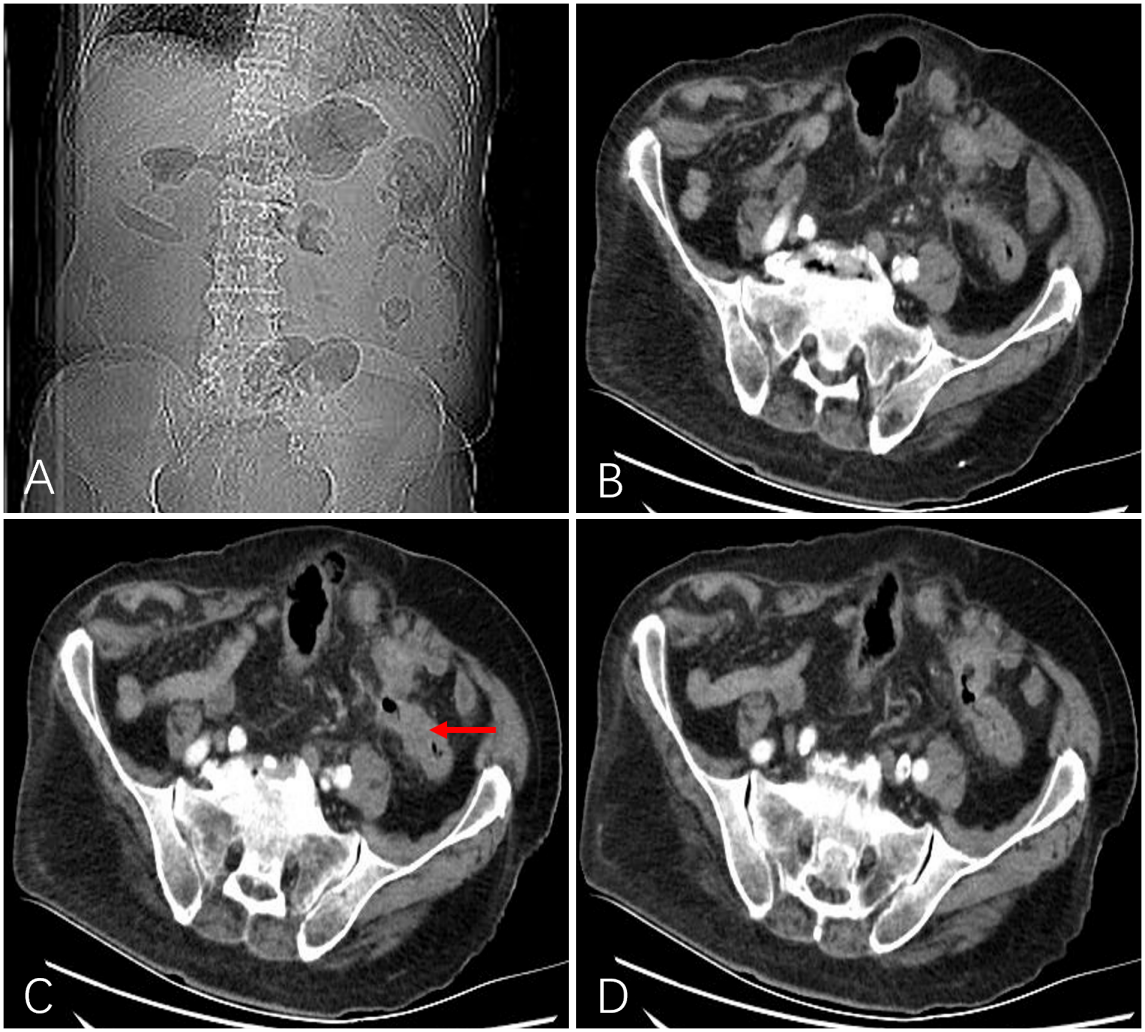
After 1 month, a follow-up abdominal X-ray showed a marked improvement in intestinal gas and fluid accumulation (A). Computed tomography showed that intestinal obstruction was relieved, the lumen was unobstructed, the wall became thinner than before (indicated by the red arrow), and the enhancement was significantly weakened (B-D).
